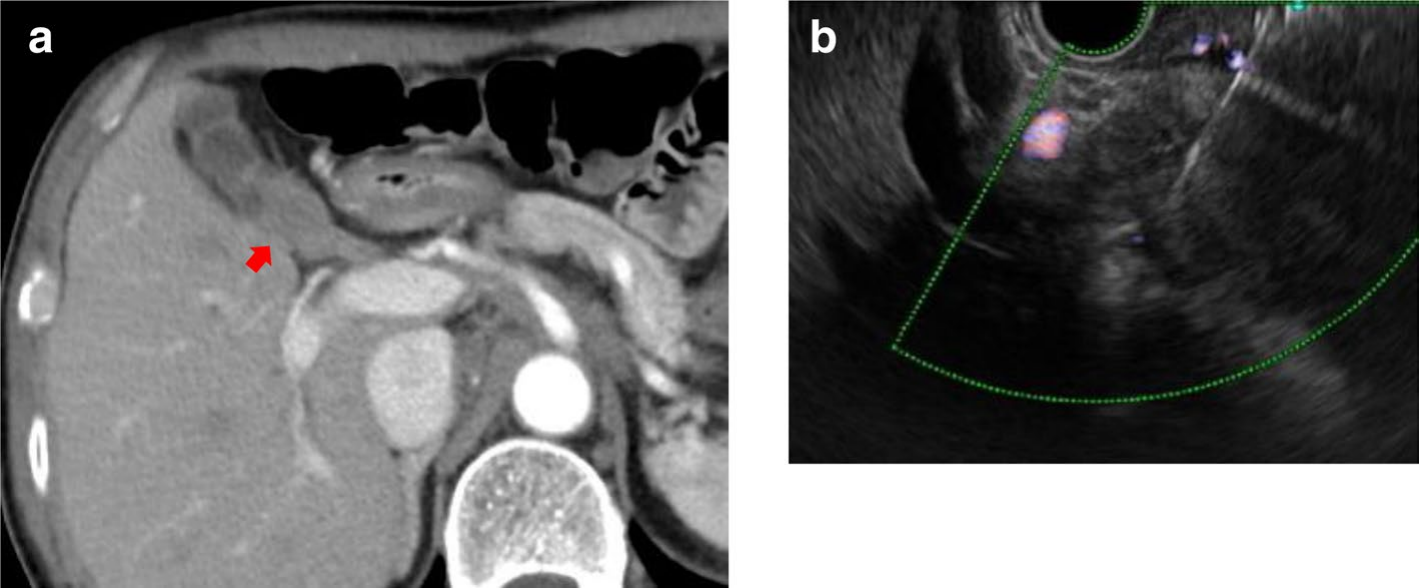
EUS-FNA for a gallbladder lesions. a CT scan shows a gallbladder lesion in the gallbladder neck (arrow). b EUS-guided FNA for a gallbladder mass lesion
